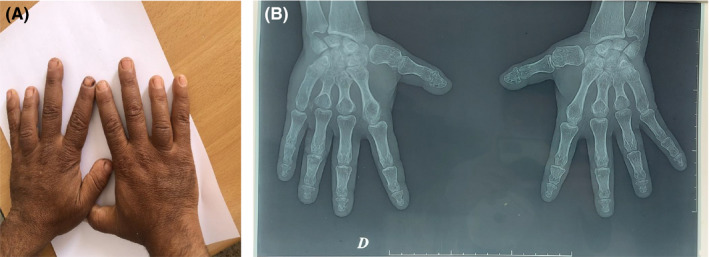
Brachydactyly. (A) Brachydactyly of the hands. Shortened fourth digits in both hands are very remarkable. (B) Radiograph of both hands: brachydactyly due to shortening of metacarpals
